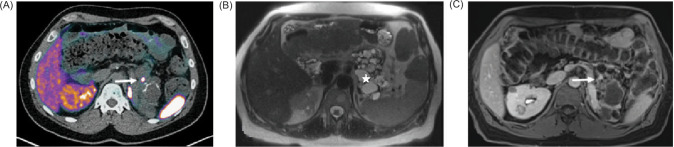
(A) Axial view on Gallium-68 Dotatate PET/CT demonstrates a focal uptake in the pancreatic tail (arrow); (B) Axial view on T-2 weighted HASTE sequence (Half Fourier Single-shot Turbo spin-Echo) demonstrates innumerable cysts within the pancreatic body and tail (star) consistent with the patient history of VHL; (C) Axial view on T-1 weighted VIBE post contrast sequence (Volumetric interpolated breath-hold examination) demonstrates multiple cysts and a focal enhancement in the pancreatic tail, corresponding to the focal radiotracer uptake, most consistent with a small neuroendocrine tumor (arrow).
